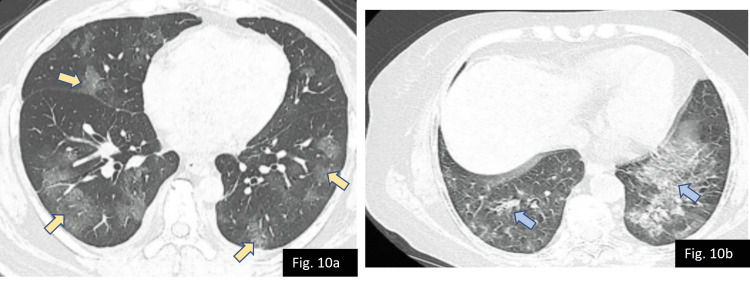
Findings within lungs at different stages of COVID-19 Image on the left (10a) shows the CT with yellow arrows pointing to areas of consolidation and in the image on the right (10b) they are indicated by a blue arrow in the CT. COVID-19: Coronavirus disease 2019 Image source: Reference no. [25]
